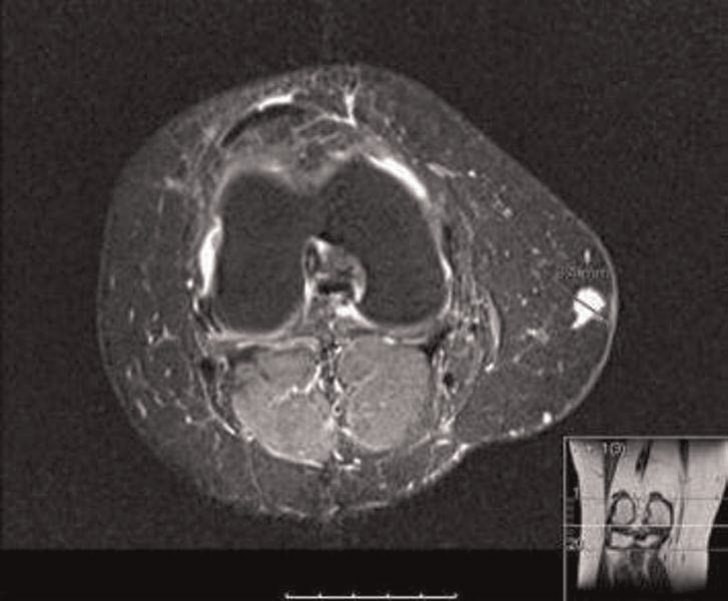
MRI scan showing angioleiomyoma in the right knee.
